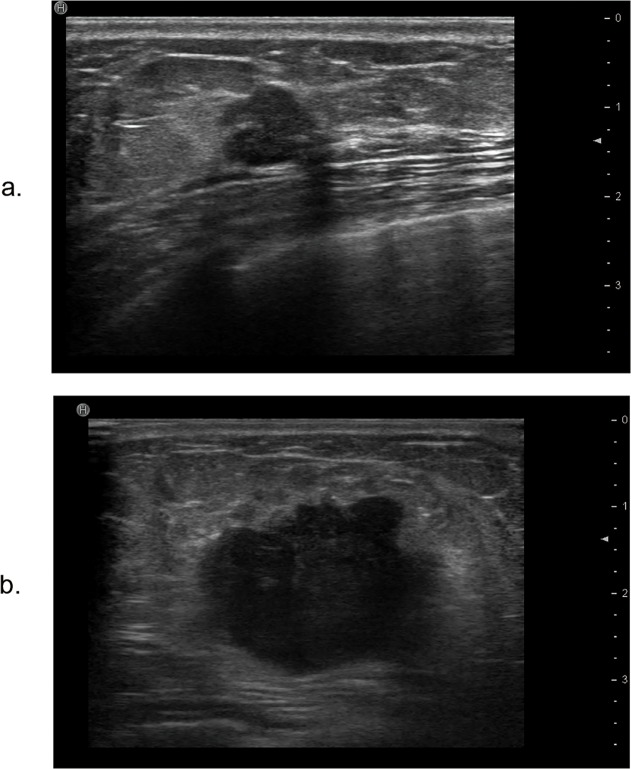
The maximum diameter of the tumor and lateral acoustic shadow feature on ultrasound. a. The maximum diameter of the normal-like breast cancer is 14 mm, and the lateral acoustic shadow is shown. b. The maximum diameter of the basal-like breast cancer is 30 mm. There is no lateral acoustic shadow.
